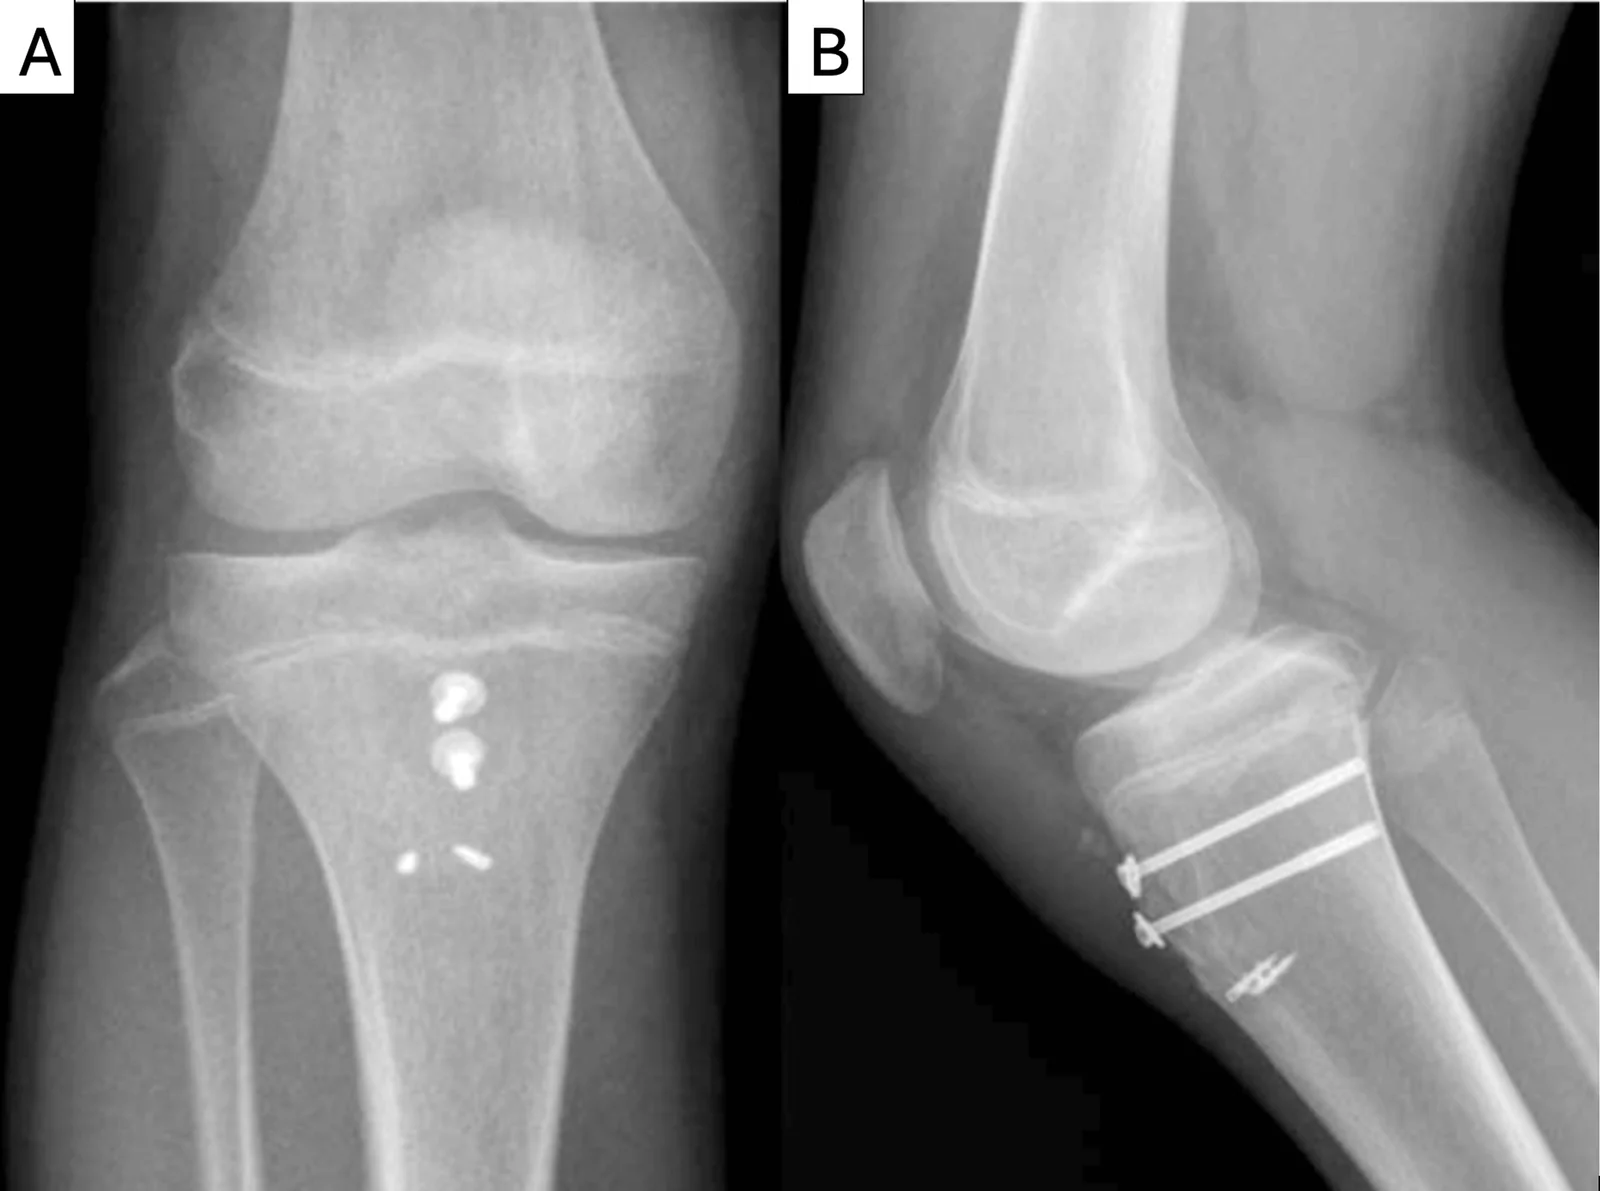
Postoperative anteroposterior and lateral radiographs of case 1. (A) Anteroposterior and (B) lateral radiographs obtained four months postoperatively, demonstrating maintained reduction, stable fixation, and fracture union following open reduction and internal fixation of the tibial tubercle with concomitant patellar tendon repair.
